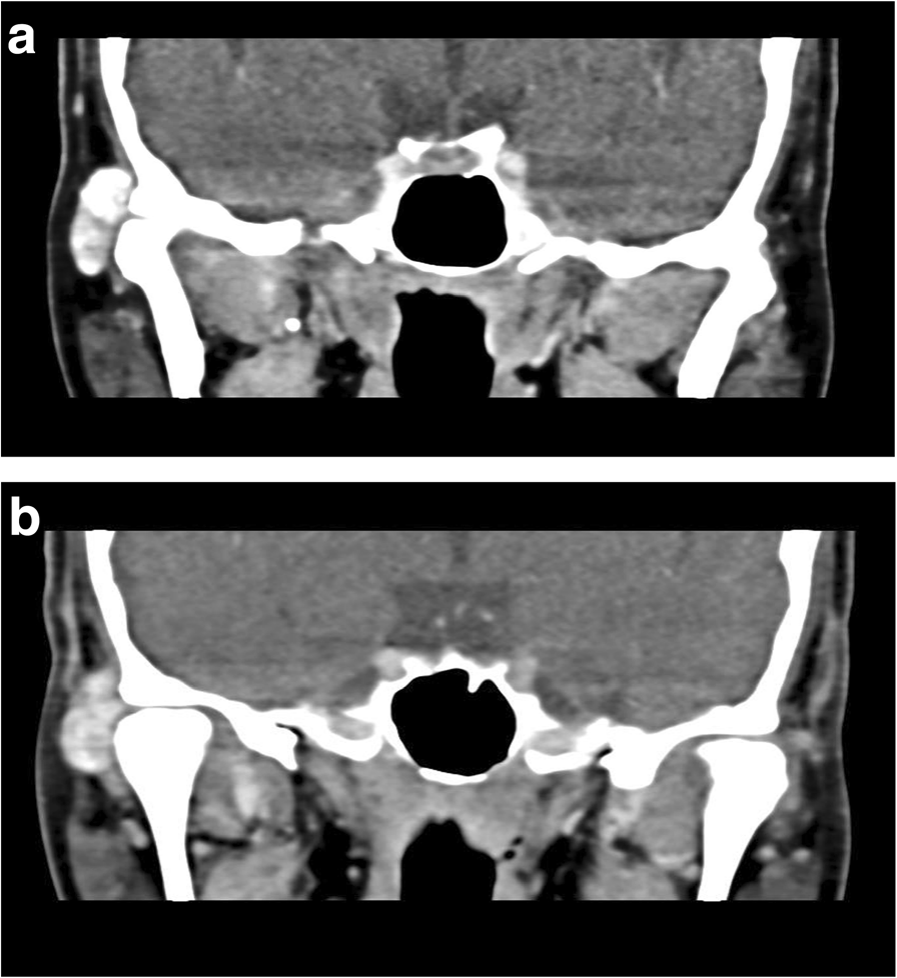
a. CT series coronal cut (soft tissue window), showing close association with lateral aspect of right condylar head. b. CT series coronal cut (soft tissue window), showing no bony erosion at right TMJ
